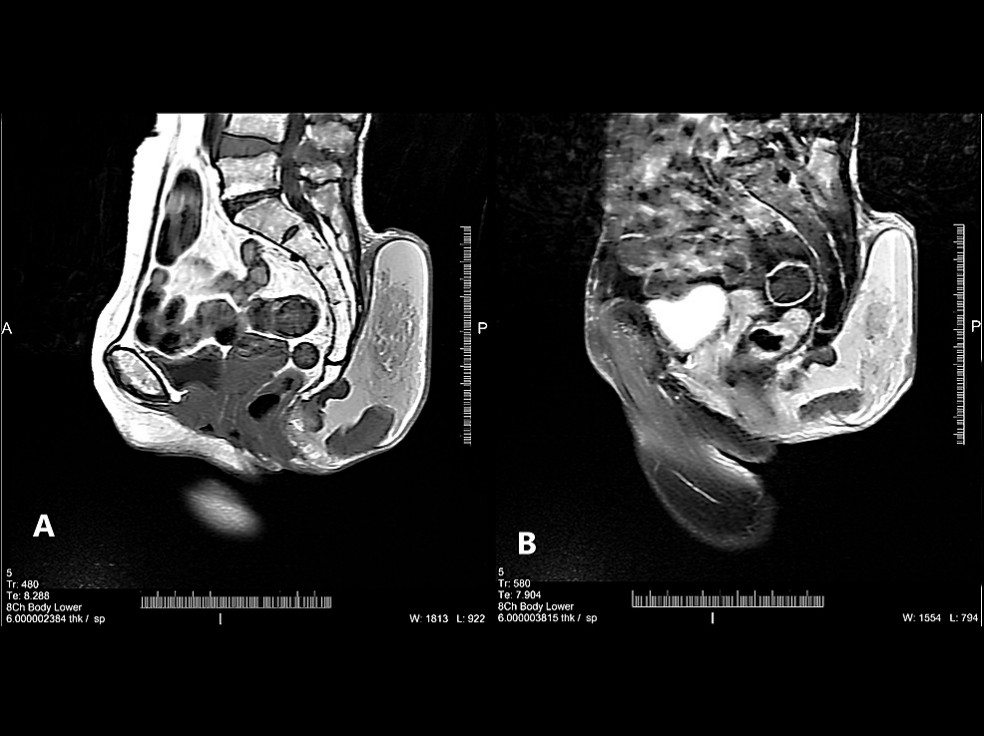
Subcutaneous sacrococcygeal ependymoma CT sagittal view (CT plain and CT contrast). (A) A large well-defined lesion is seen involving the sacrococcygeal region in the skin subcutaneous plane. Anteriorly, the lesion abutting coccyx vertebral body with no bony erosion seen (image plane sagittal T1 study). (B) Heterogenous enhancement on post-contrast images with multiple non-enhancing areas. The lesion shows the surrounding T2W1 hypointense capsule.
